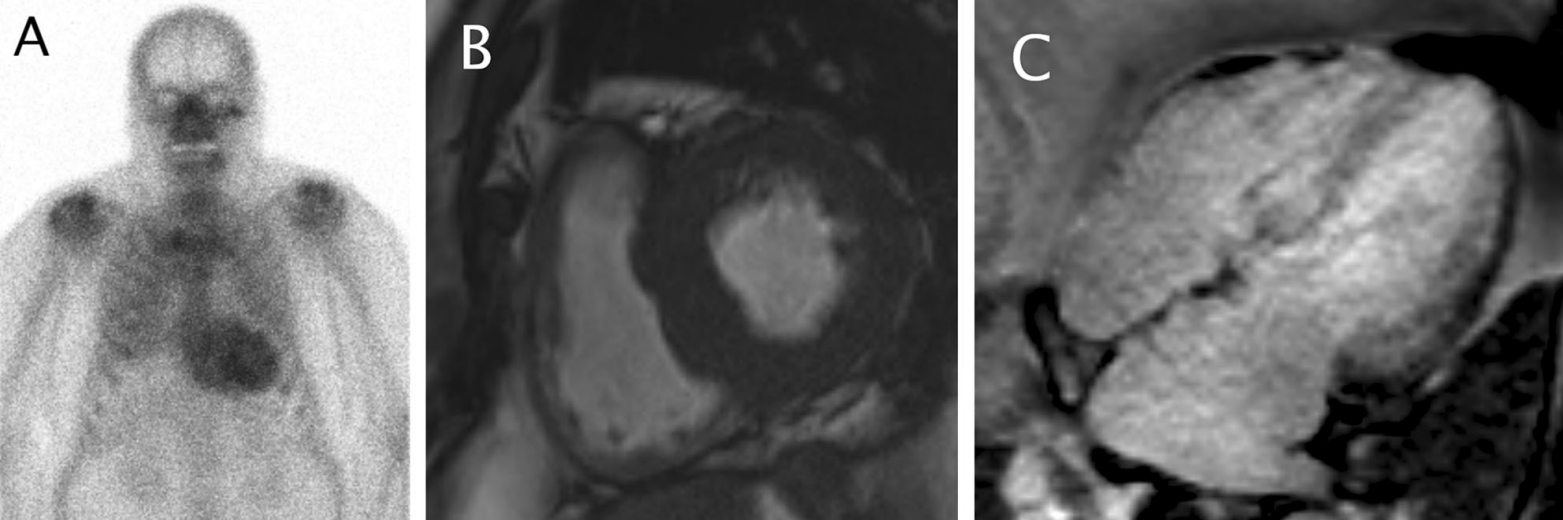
Incidental cardiac and pulmonary uptake on bone scintigraphy imaged to exclude prostate cancer bone metastases. Heart-to-contralateral ratio (H/CL) was 1.83. (A) After 5 years the same patient was evaluated in cardiology department for suspected chronic heart failure and left ventricular hypertrophy. Magnetic resonance imaging demonstrated thick septum of 22 mm (B) and wide-spread late gadolinium enhancement characteristic of cardiac amyloidosis (C). Laboratory testing for light chain disease was negative and diagnosis of ATTR cardiomyopathy was made
